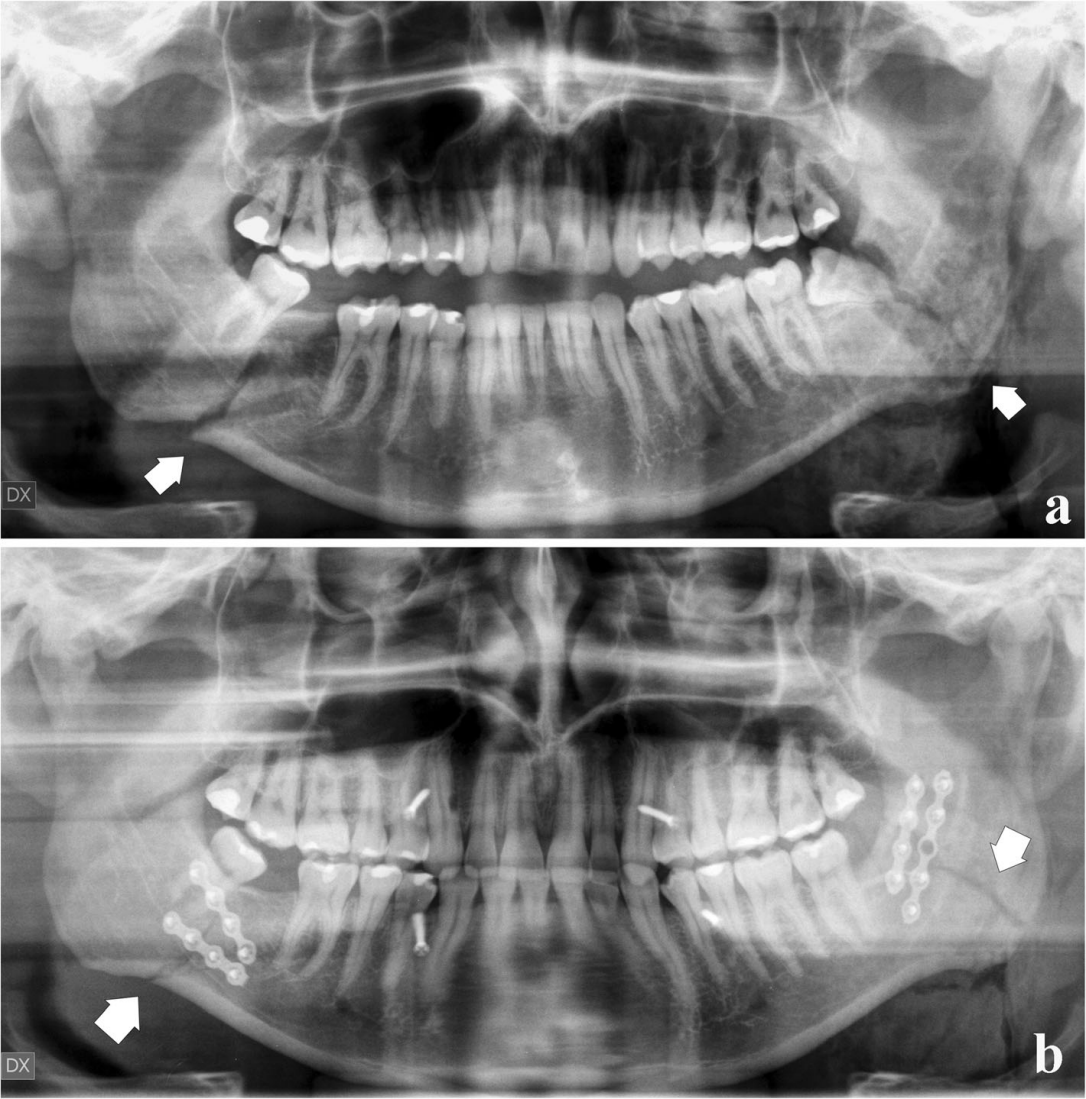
Bilateral angle fracture. a Panoramic radiograph shows fractures of both mandibular angles (arrows) involving the lower third molars. b After removing the lower left third molar, rigid internal fixations with osteosynthesis plates merge the bone segments
